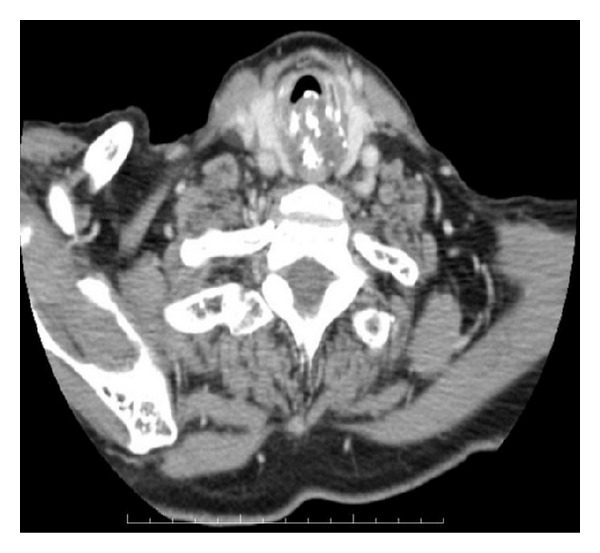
CT imaging. A mass that has popcorn calcification is seen in the posterior part of laryngeal airway.
